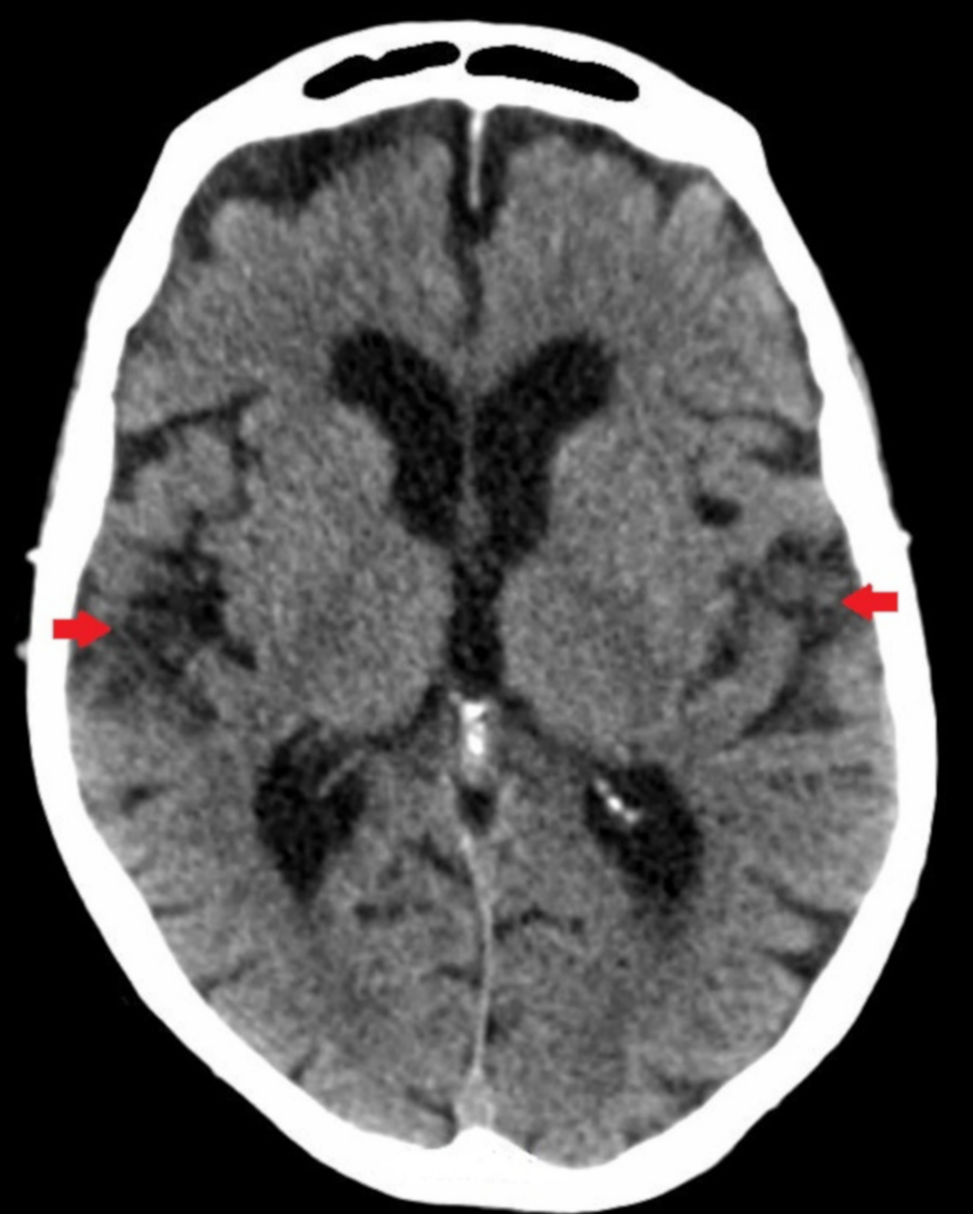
Computed tomography scan of the brain demonstrating mild parietotemporal atrophy.
